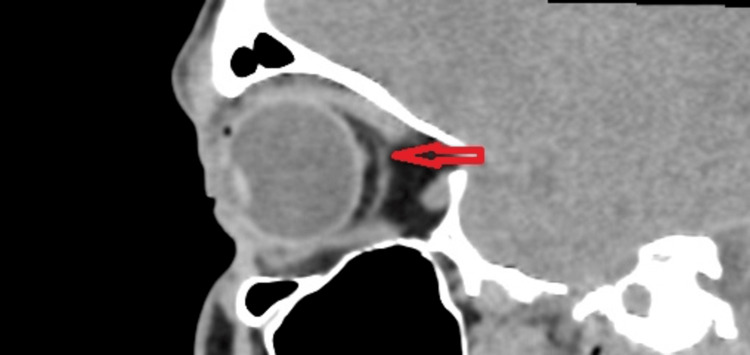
Saggital view of a plain computed tomography showing the orbit of the patient with an elongated tubular structure seen (red arrow) just behind the globe, extending from the superior to inferior rectus muscles
